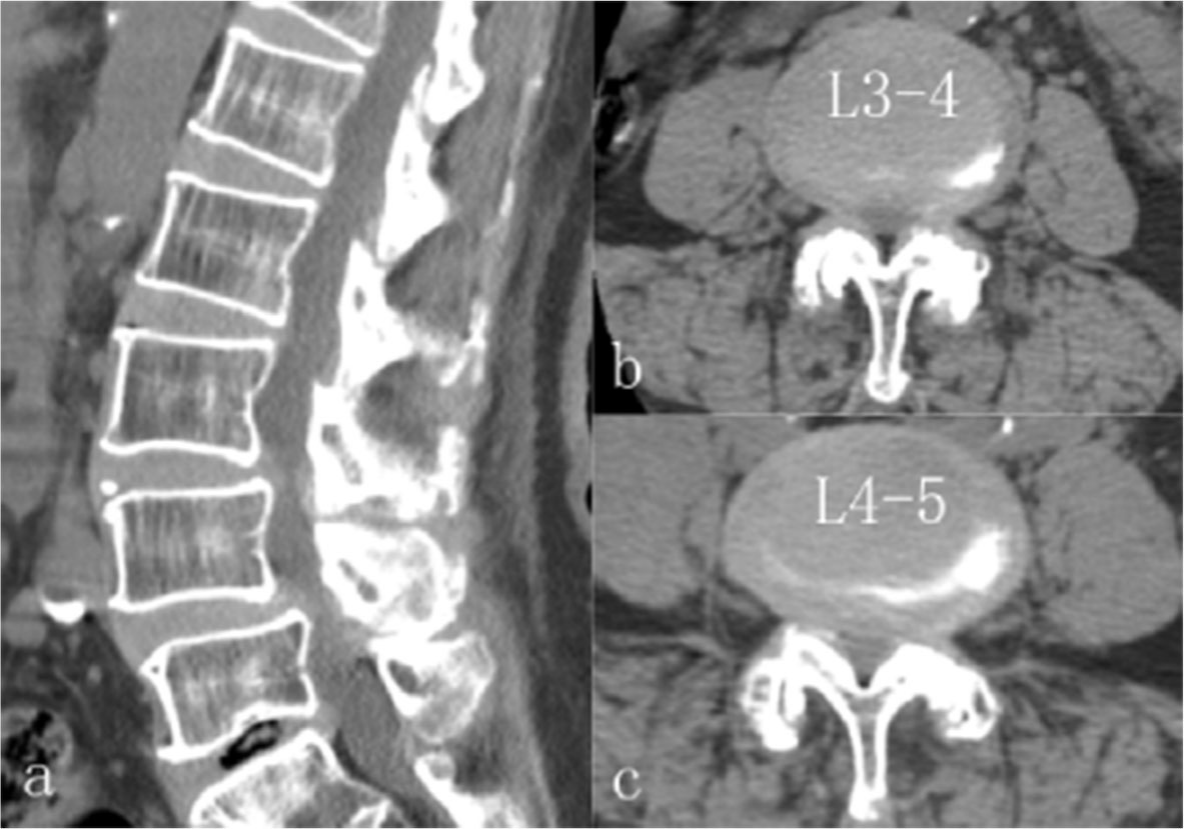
CT examination revealed degenerative spondylolisthesis at L4; moreover, LDH at L3–4 and L4–5, the latter was more severe, especially on left side
